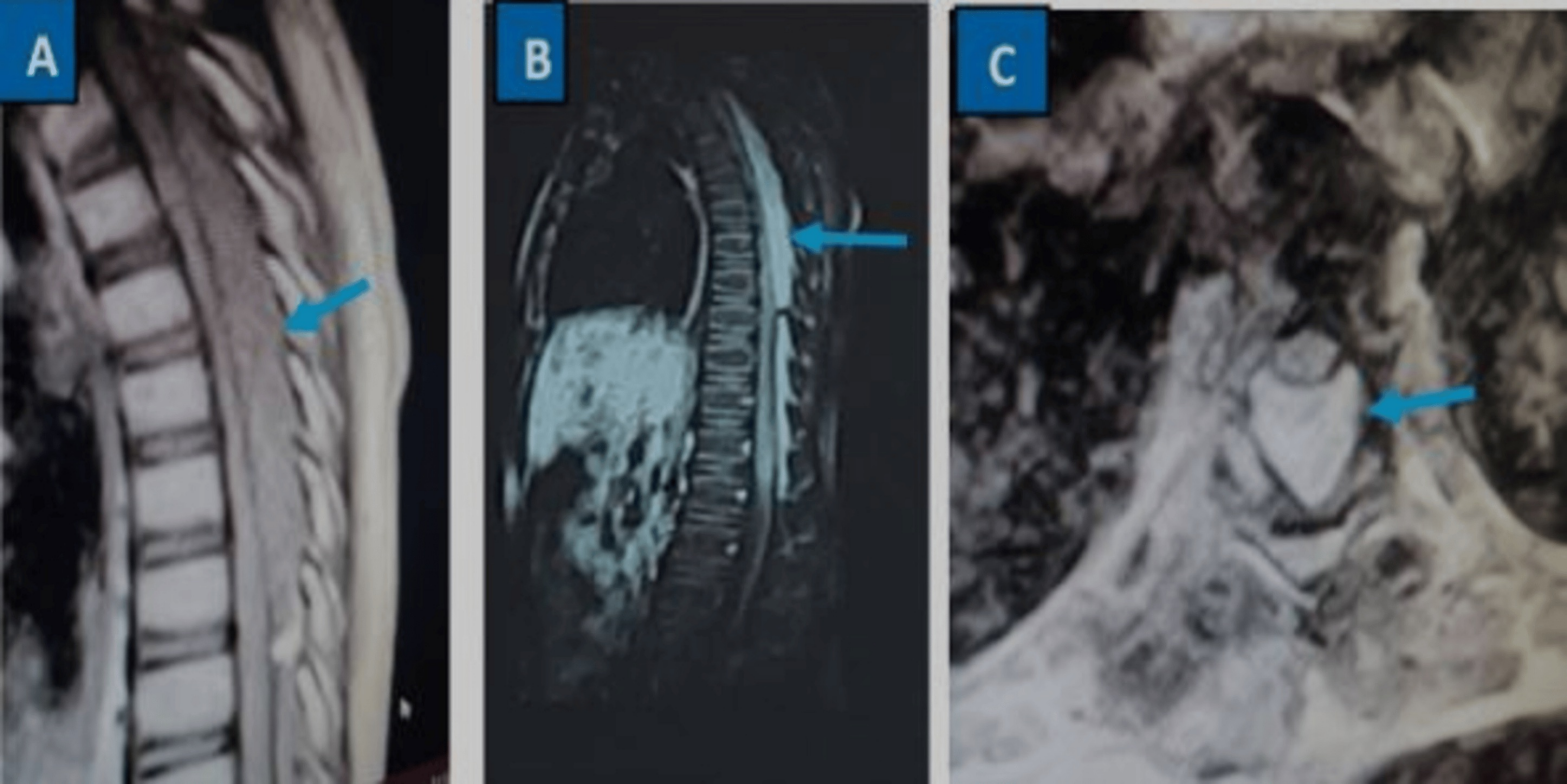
MRI of the patient's thoracolumbar spine A: Sagittal MRI (T1-weighted) shows a posterior, extra-medullary, intracanal mass extending from T1 to T10. B: Sagittal MRI (T2-weighted) displays a posterior, extra-medullary, intracanal mass extending from T1 to T10. The MRI signal characteristics suggest a compressive, fatty signal mass (blue arrow). C: Axial MRI (T2-weighted) reveals a posterior, extra-medullary, intracanal mass compressing the spinal cord (blue arrow).
